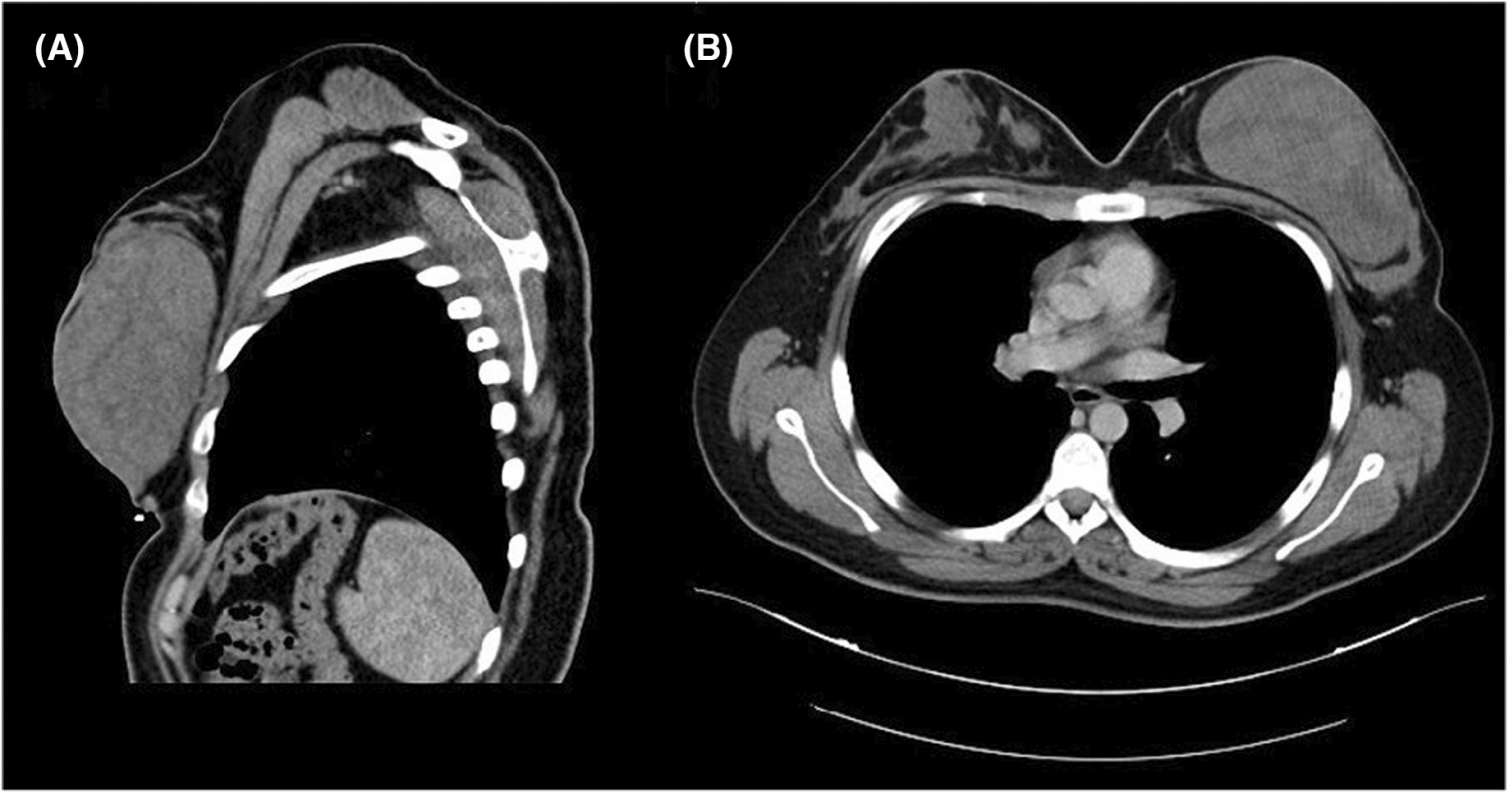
(A) Sagittal CT scan showing a large well‐circumscribed rounded mass measuring (13 × 8) cm occupying most of the left breast; (B) Axial CT section showing two small well‐defined nodular lesions in the right breast with a large mass in the left breast.
